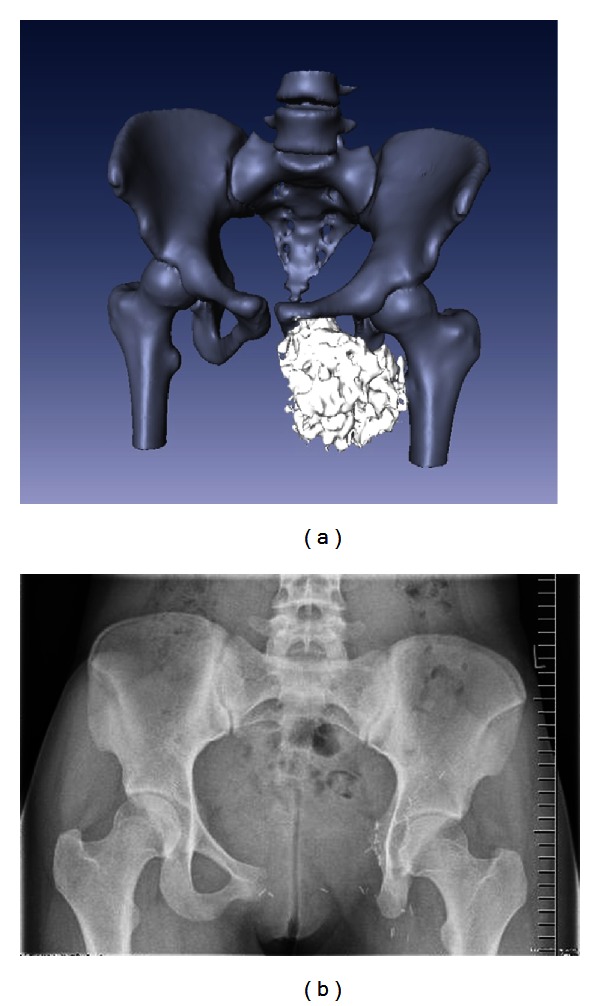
(a) CT reconstruction of the pelvis of a 15-year-old girl with a chondrosarcoma of the left os pubis and os ischii. (b) Anteroposterior radiograph after P3 resection.
